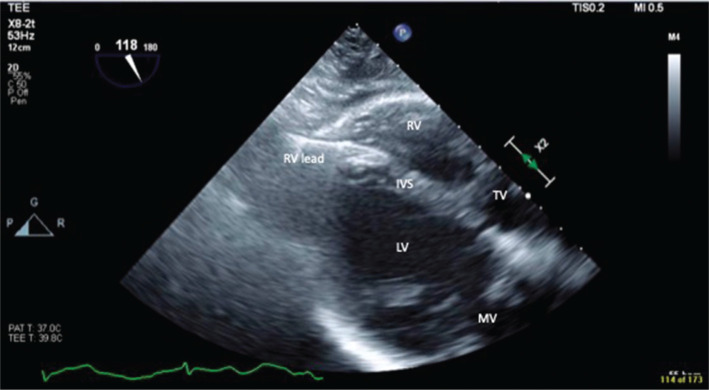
Intraoperative transesophageal echocardiography showing the right ventricular lead perforating through the apex. *Abbreviations:* IVS, interventricular septum; LV, left ventricle; MV, mitral valve; RV, right ventricle; TV, tricuspid valve.
